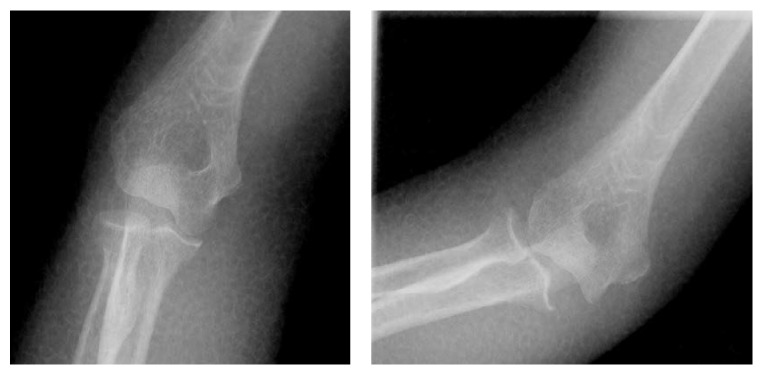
Postreduction radiographs revealed obvious varus and valgus instability of the joint. However, redislocation was not observed in the range of motion from 30° to 90°.
